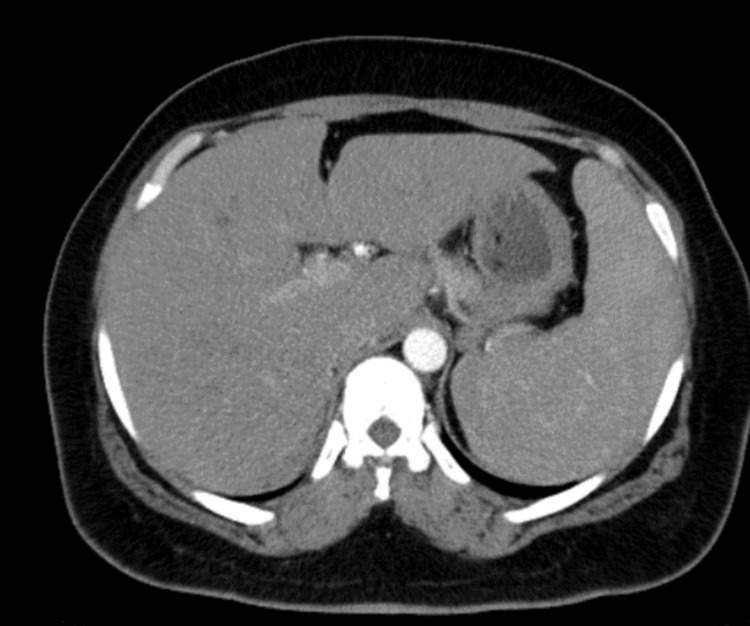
Abdominal CT scan showing hepatosplenomegaly CT: computerized tomography
